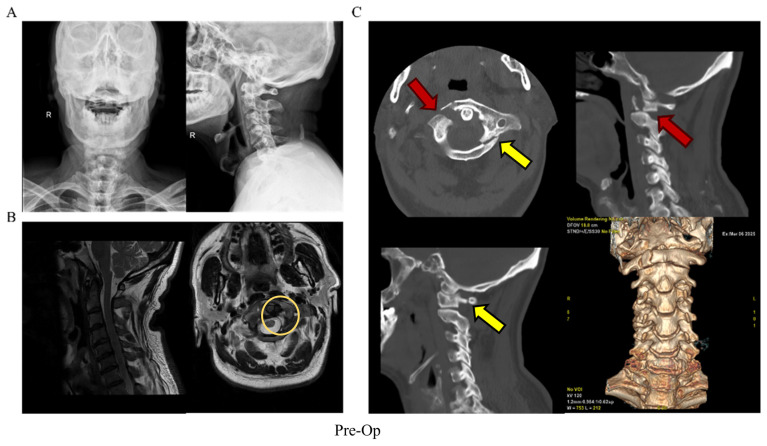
Preoperative imaging findings: (A) Anteroposterior and lateral radiographs of the cervical spine. (B) Cervical spine MRI demonstrating complete transverse atlantal ligament (TAL) rupture (yellow circle), mild cervical intervertebral disk protrusion, and no spinal cord compression. (C) Cervical spine CT showing a displaced fracture at the transitional region between the right anterior arch and lateral mass of the atlas combined with atlantoaxial dislocation (red arrow), as well as a fracture of the left posterior arch (yellow arrow).
